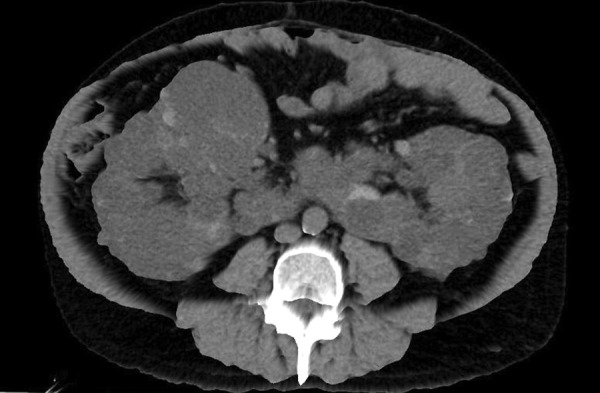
3D reconstruction of computed tomography image showing renal fusion and massive cysts; axial section.
